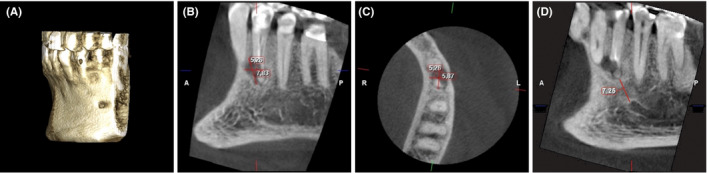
A, CBCT three‐dimensional image, B, C, extension of mesiobuccal radiolucency in the sagittal and axial images, and D, the distance from root apex of tooth #34 to mental foramen in sagittal CBCT image
